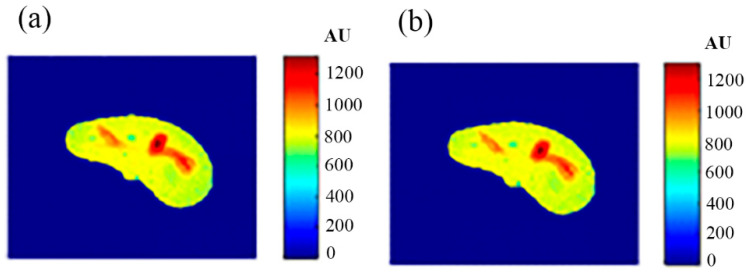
(a) The real MR image, and (b) the generated MR image using *T_E_* and *T_R_* of 20 ms and 800 ms, respectively.
